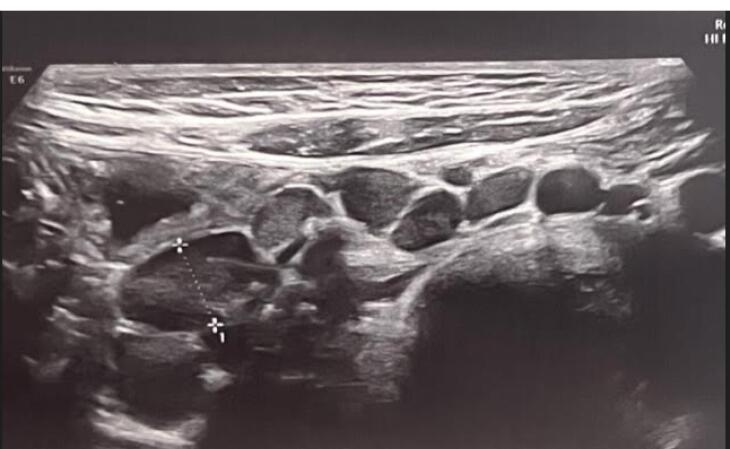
As in our study, mesenteric lymphadenitis was the most common abdominal finding in 60% of cases with COVID-19 disease which was similar to previous studies. Considering previous studies, it has been shown that imaging studies, such as abdominal ultrasound or even abdominal computed tomography (CT), may simplify differentiating true surgical emergencies in questioned cases of the acute abdomen.^34^ In our study, abdominal ultrasound was practical in cases with abdominal pain, and similar to other studies, surgically confirmed cases of acute appendicitis were found in patients with COVID-19 in our investigation.^38-42^ However, in a few studies, non-operative management of uncomplicated acute appendicitis accompanying COVID-19 infection with antibiotics has been documented.^43^
